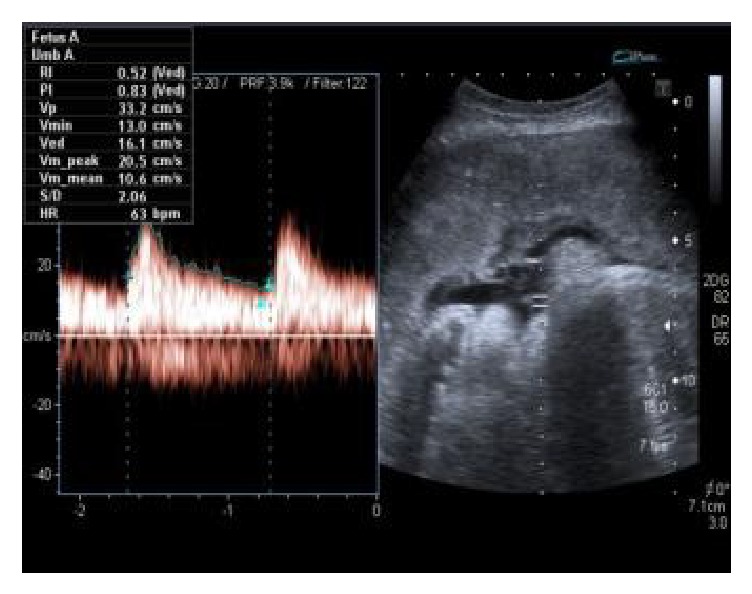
(Umbilical artery Doppler. Good EDF, PI 0.83).
